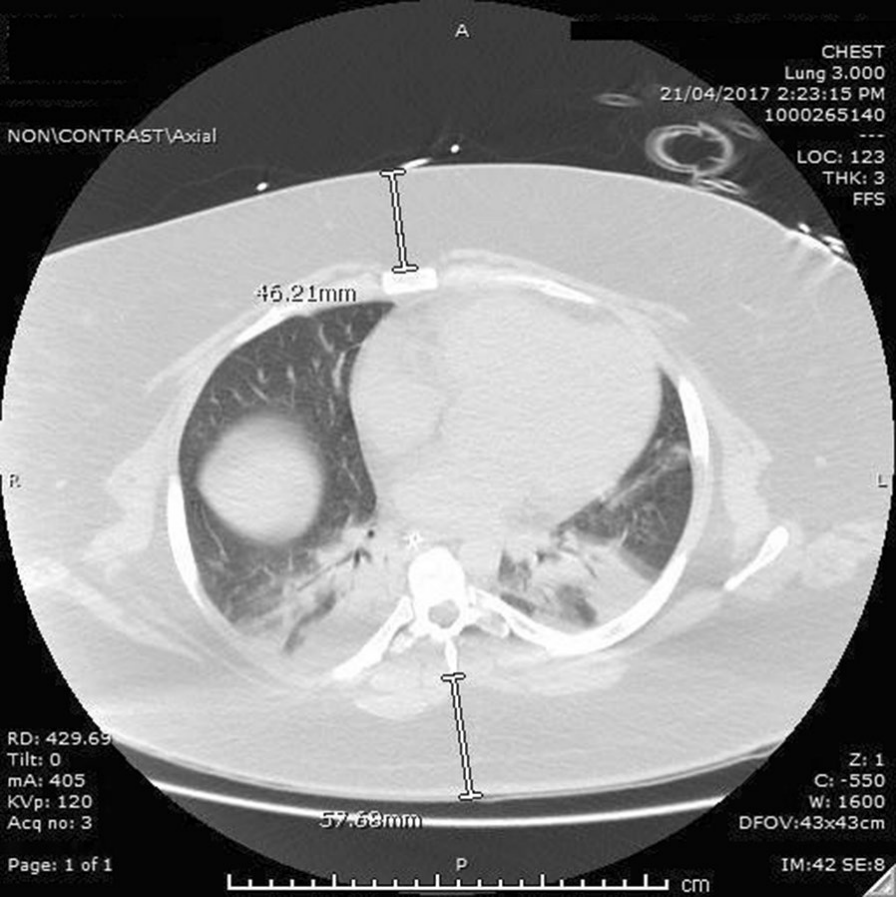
Example CT thorax image demonstrating sites at which measurement of SAT were made in the anterior–posterior plane
